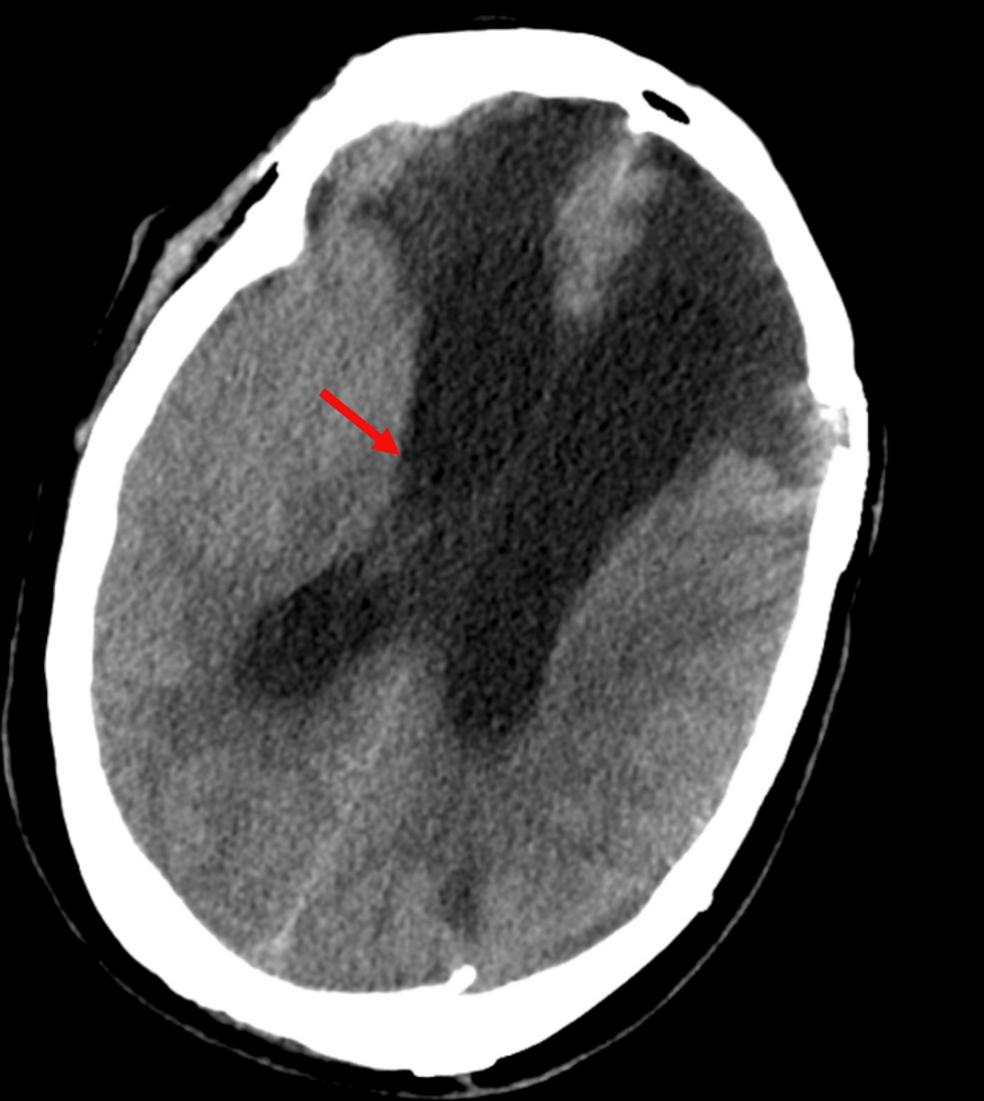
Axial CT head. The view shows post-traumatic hydrocephalus (red arrow).
